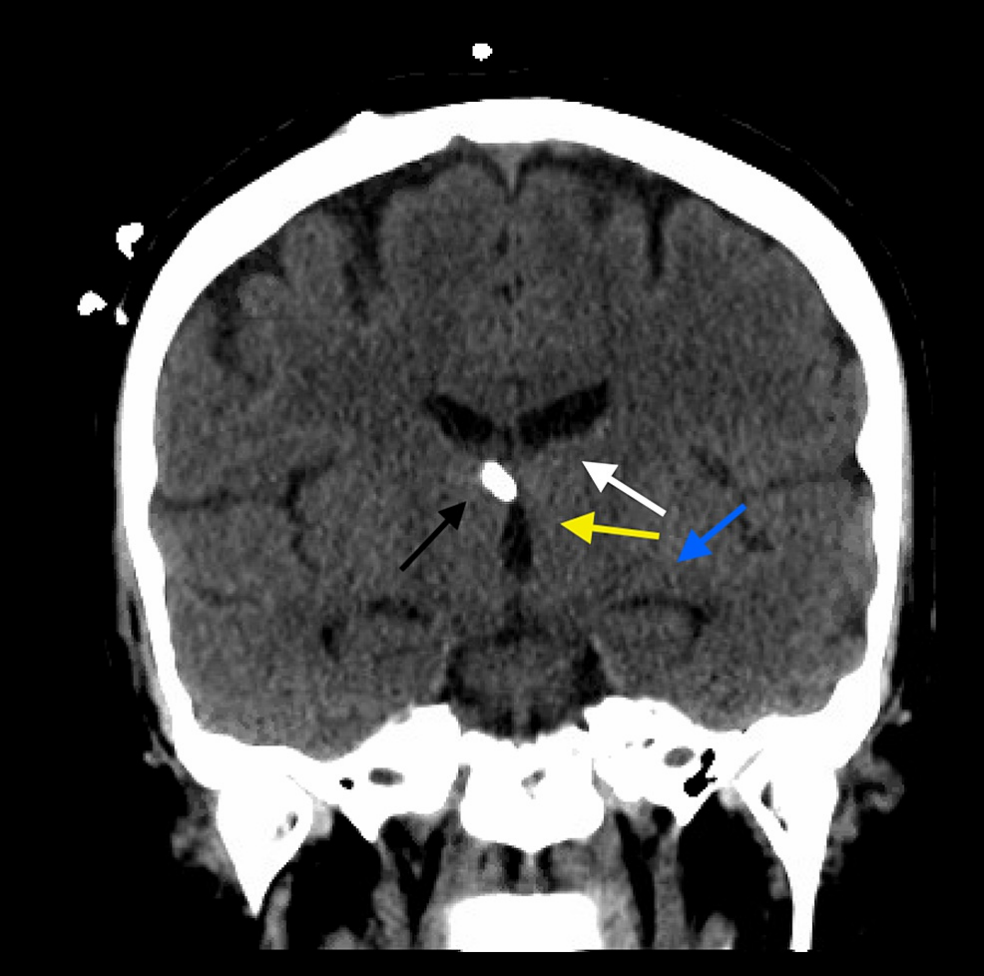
Coronal non-contrasted CT head demonstrating interval decrease in the size of lateral ventricle (white arrow), third ventricle (yellow arrow), and temporal horns (blue arrow) following functioning EVD lowered to 0 cm H2O terminating in right foramen of Monro (black arrow). CT, computed tomography; EVD, external ventricular drain.
